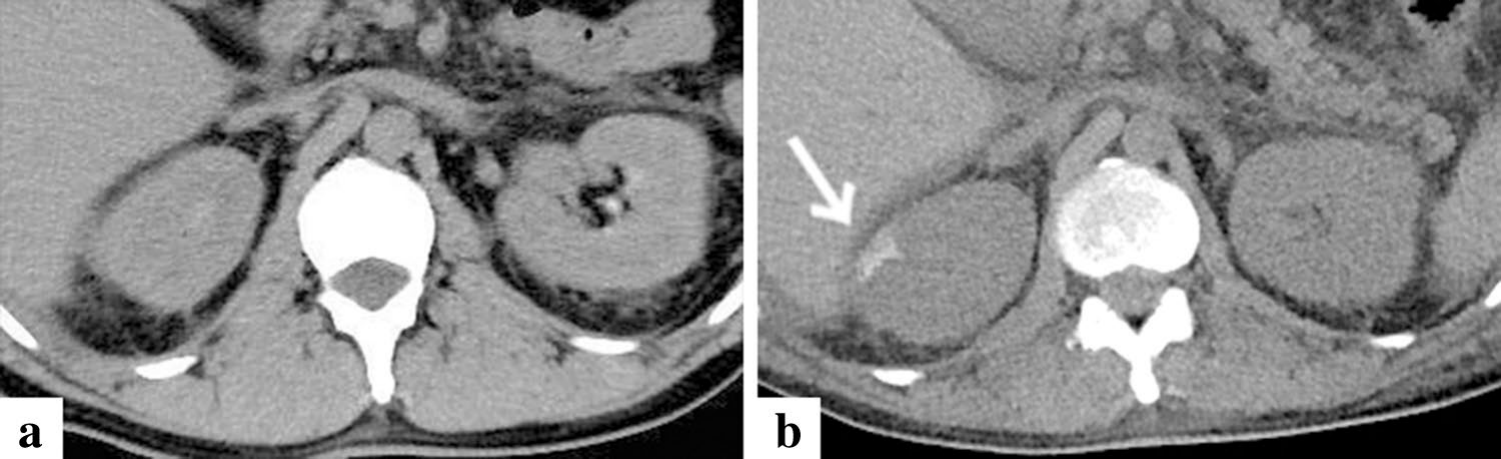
Representative case of renal calcification in a 50-year-old patient (case no. 2). Computed tomography (CT) imaging on day 41 since the onset of symptoms (a) and after one month of the admission (b). CT imaging on admission showed fatty tissue turbidity around the bilateral kidneys due to acute renal injury from TAFRO syndrome (a). One month after admission, CT imaging showed improvement in the finding of fatty tissue turbidity around the bilateral kidneys; however, calcification appeared in the kidney (b, white arrow)
